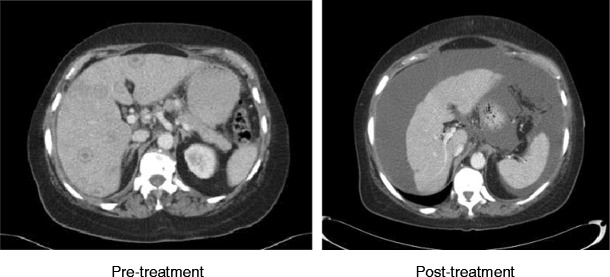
Post-treatment CT shows a small liver with nodular contour on a background of ascites but with a normal-sized spleen. The patient had completed chemotherapy for metastatic breast carcinoma with multiple hepatic metastases. The sites of retraction correspond to sites of tumour on the pre-treatment CT
